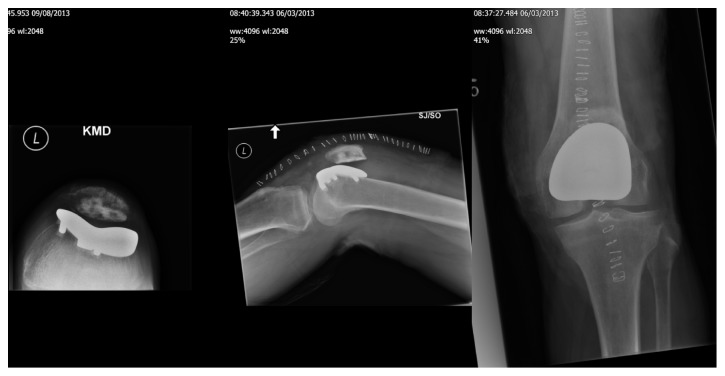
Postoperative radiograph of patellofemoral joint replacement.
